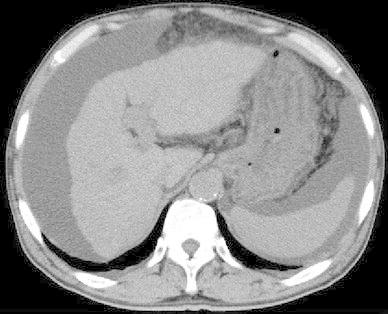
Plain abdominal computed tomography image. The hepatic lobes are atrophic with a blunt margin and irregularity on the surface. A moderate volume of ascites retention is also observed
